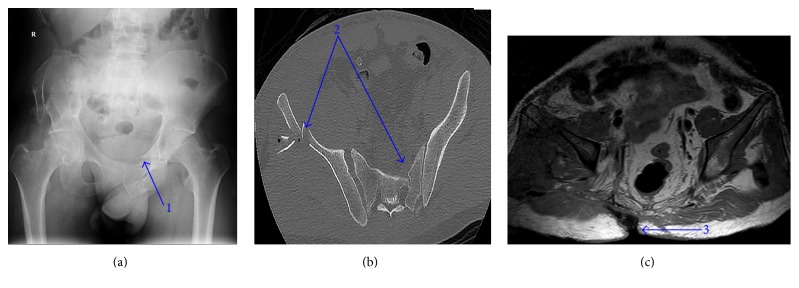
(a) X-ray image of the pelvic fracture four months after trauma (1); (b) Computed Tomography in the fifth day from admission revealing the fracture of the pelvis (2); (c) magnetic resonance imaging 40 days from admission demonstrating the remnant of the perineal wound defect (3).
